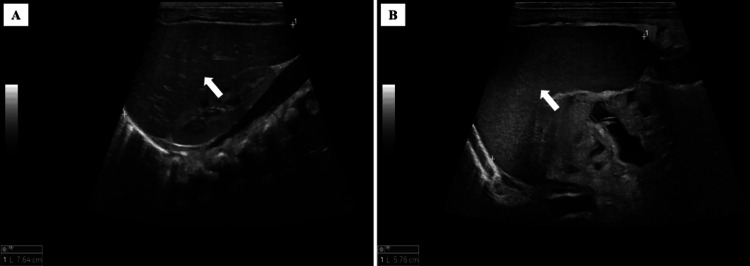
Abdominal US images of a patient with trichohepatoenteric syndrome (Patient 1) US: ultrasound (A) US image showing hepatomegaly with altered parenchymal echogenicity (arrow); (B) US image showing splenomegaly with altered parenchymal echogenicity (arrow)
